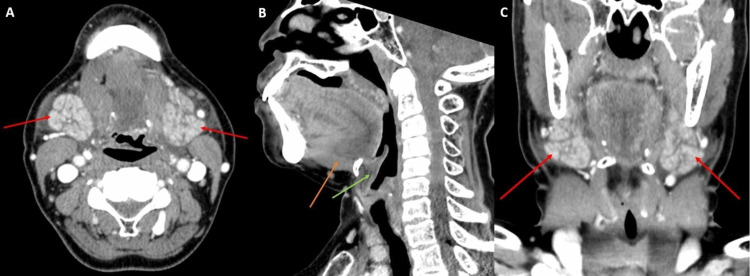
Axial, sagittal, and coronal enhanced CT A: Axial enhanced CT demonstrates submandibular gland swelling (red arrows). B: Sagittal enhanced CT demonstrates pre-epiglottic fat stranding (green arrow) and base of tongue swelling (orange arrow). C: Coronal enhanced CT demonstrates submandibular gland and surrounding submandibular space swelling (red arrows) CT: computed tomography
